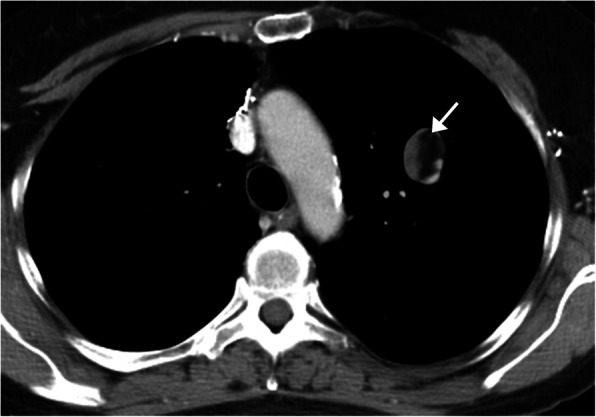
Hamartoma. CT chest with contrast demonstrating the pathognomonic finding of intrinsic fat within a pulmonary hamartoma of the left upper lobe (arrow)
